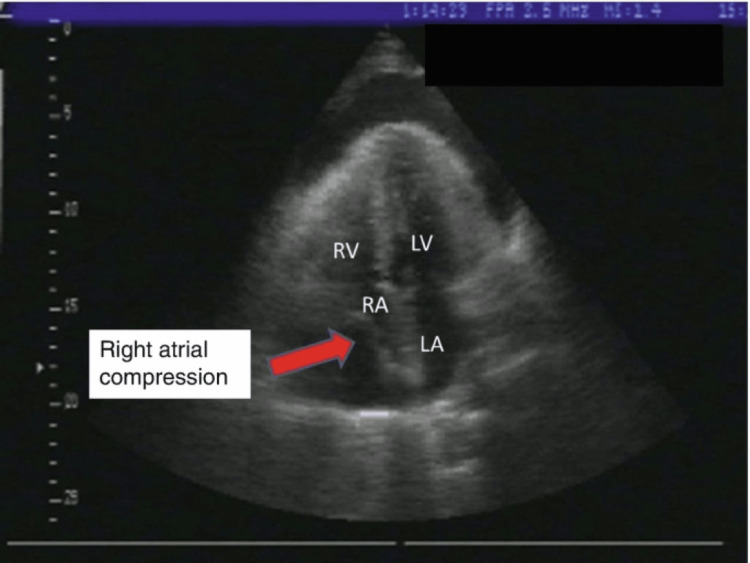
Example of RA compression on TEE via mid-esophageal four-chamber view RA: right atrial; TEE: transesophageal echocardiography
